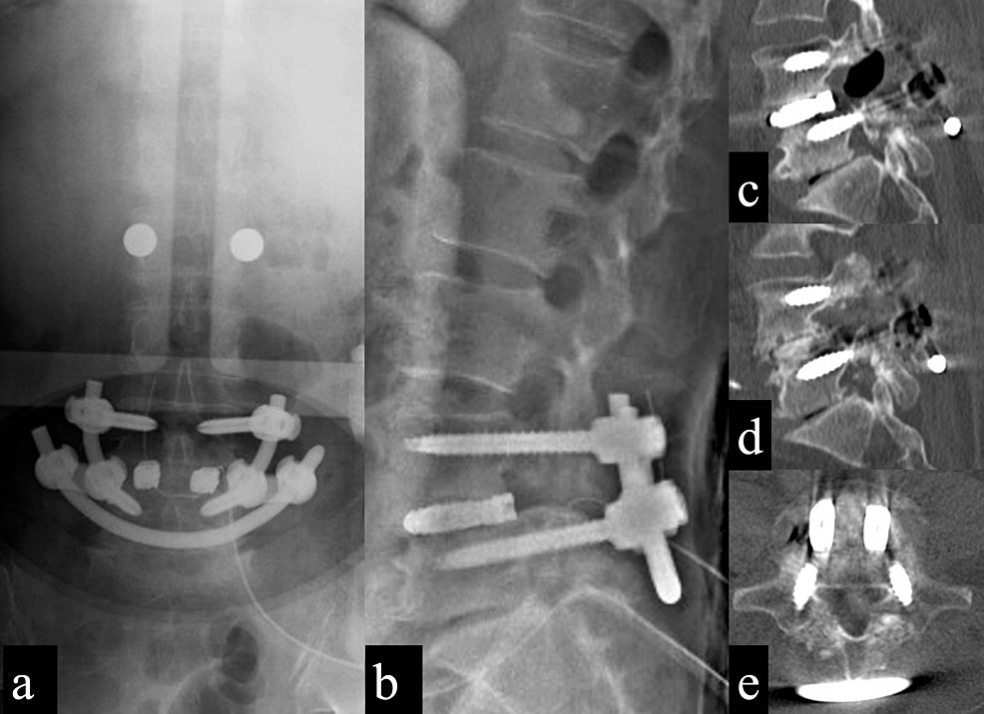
Imaging findings after transforaminal lumbar interbody fusion (TLIF) of L4/5 and smiley face rod (SFR) of L5 2a: A plain AP X-ray image of the lumbosacral spine shows TLIF of L4/5 and SFR of L5 performed using dual-headed pedicle screws. 2b: A plain lateral X-ray image of the lumbosacral spine shows a reduction of L4 anterior slip. 2c: A plain CT sagittal view (right) shows no gap at the L5 pars cleft. 2d: A plain CT sagittal view (left) shows no gap at the L5 pars cleft. 2e: A plain CT axial view shows no gap at the L5 bilateral pars clefts.
